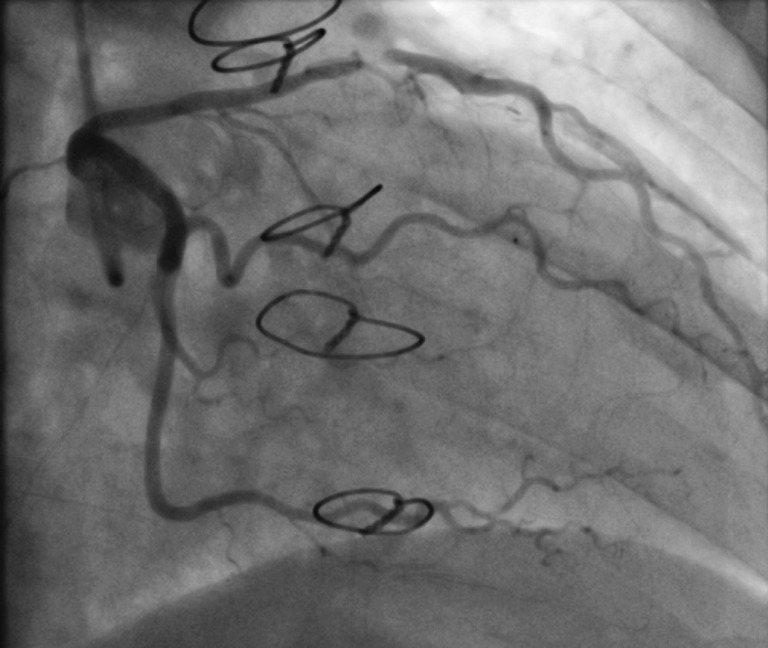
Subocclusive left anterior descending artery incidentally detected in an asymptomatic patient with anterior wall hypocontractility on routine echocardiographic exam 8 months after transplantation.
